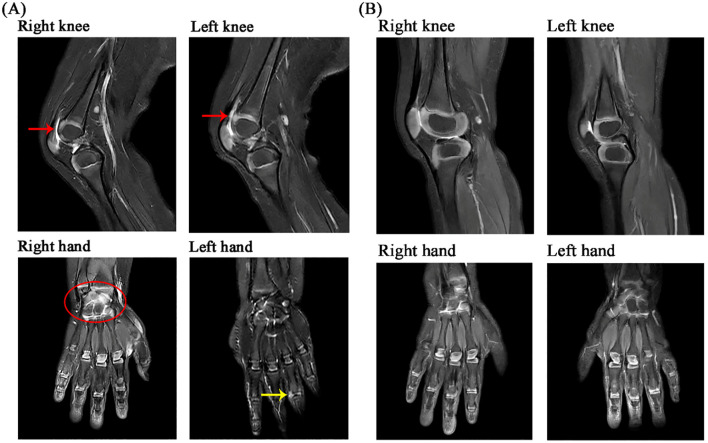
(A) MRI findings of the affected joints before TNFi treatment. Synovitis was observed in the biliteral knee (right arrow) and right wrist joints (right circle). Swelling of the soft tissue at the proximal interphalangeal joint of the fourth finger of the left hand, accompanied by tenosynovitis (yellow arrow). (B) Synovitis, extension, and swelling of the affected joints were alleviated after 6 months of TNFi treatment. MRI, magnetic resonance imaging; TNFi, tumor necrosis factor inhibitor.
